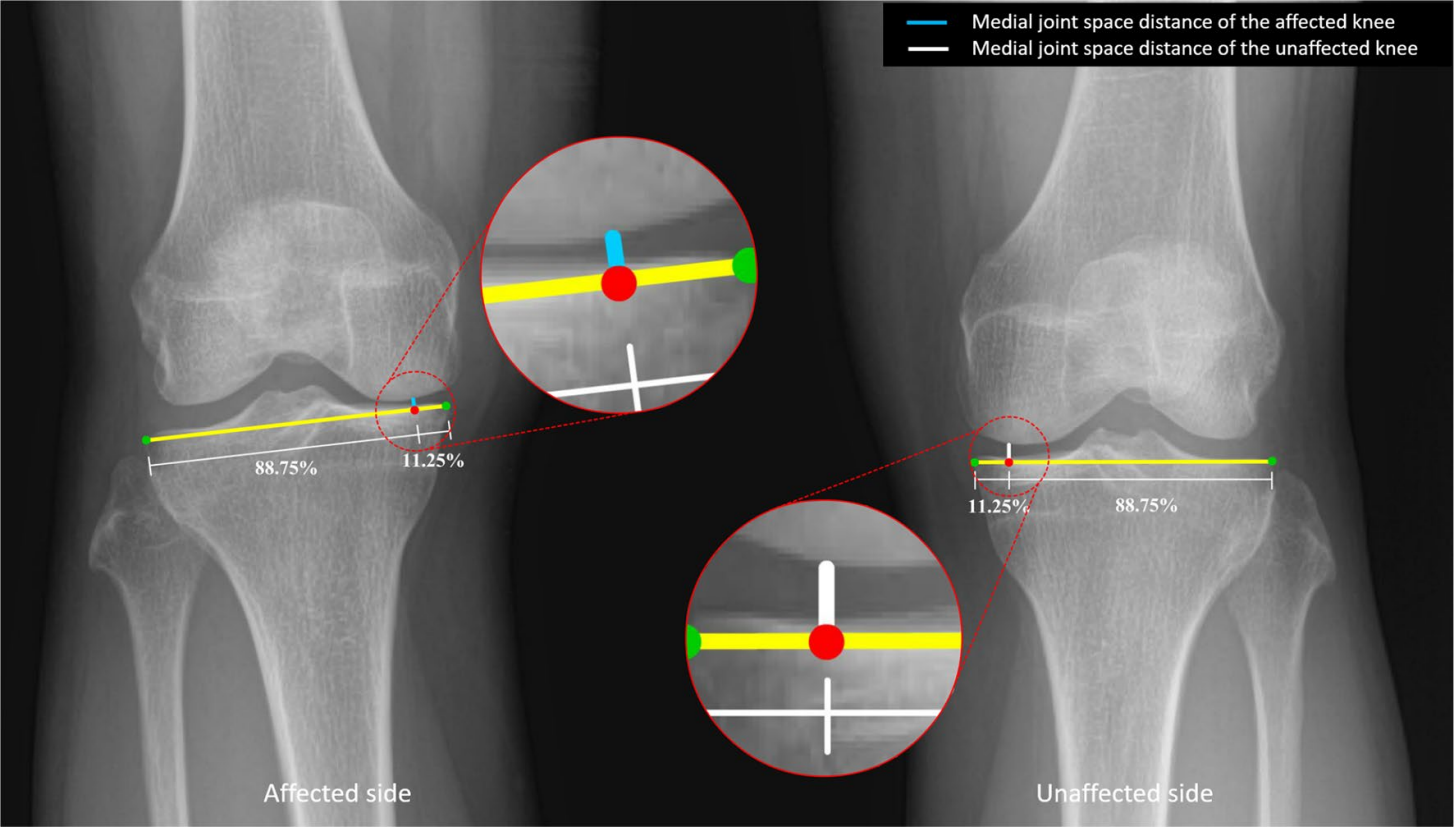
An anteroposterior radiographic image of both knees showing the peripheral medial joint space distance between the medial femoral condyle and the medial tibial plateau of the affected (blue line) and unaffected (white line) knees. The study analysis was based on the ratio of the peripheral medial joint space distance in the affected knee (A, blue line) relative to the peripheral medial joint space distance in the unaffected knee (B, white line) (A, B).
